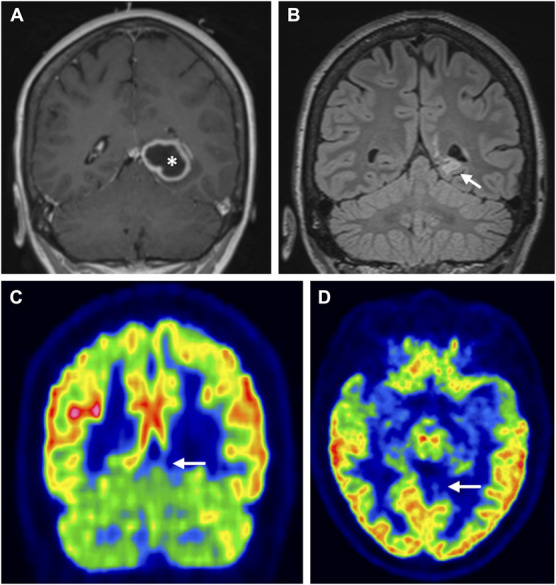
Gliosis and hypometabolism after brain abscess. A, T1-weighted postcontrast MRI showing an abscess (*) in the left precuneus before pus removal; B, T2-weighted fluid-attenuated inversion recovery MRI showing gliosis in the area of the abscess 2 years after pus removal (arrow). C and D, [^18^F]-fluoro-2-deoxy-D-glucose positron emission tomography/computed tomography (coronal and axial sections, respectively) in the same patient, showing neocortical hypometabolism (arrows) 2 years after pus removal.
